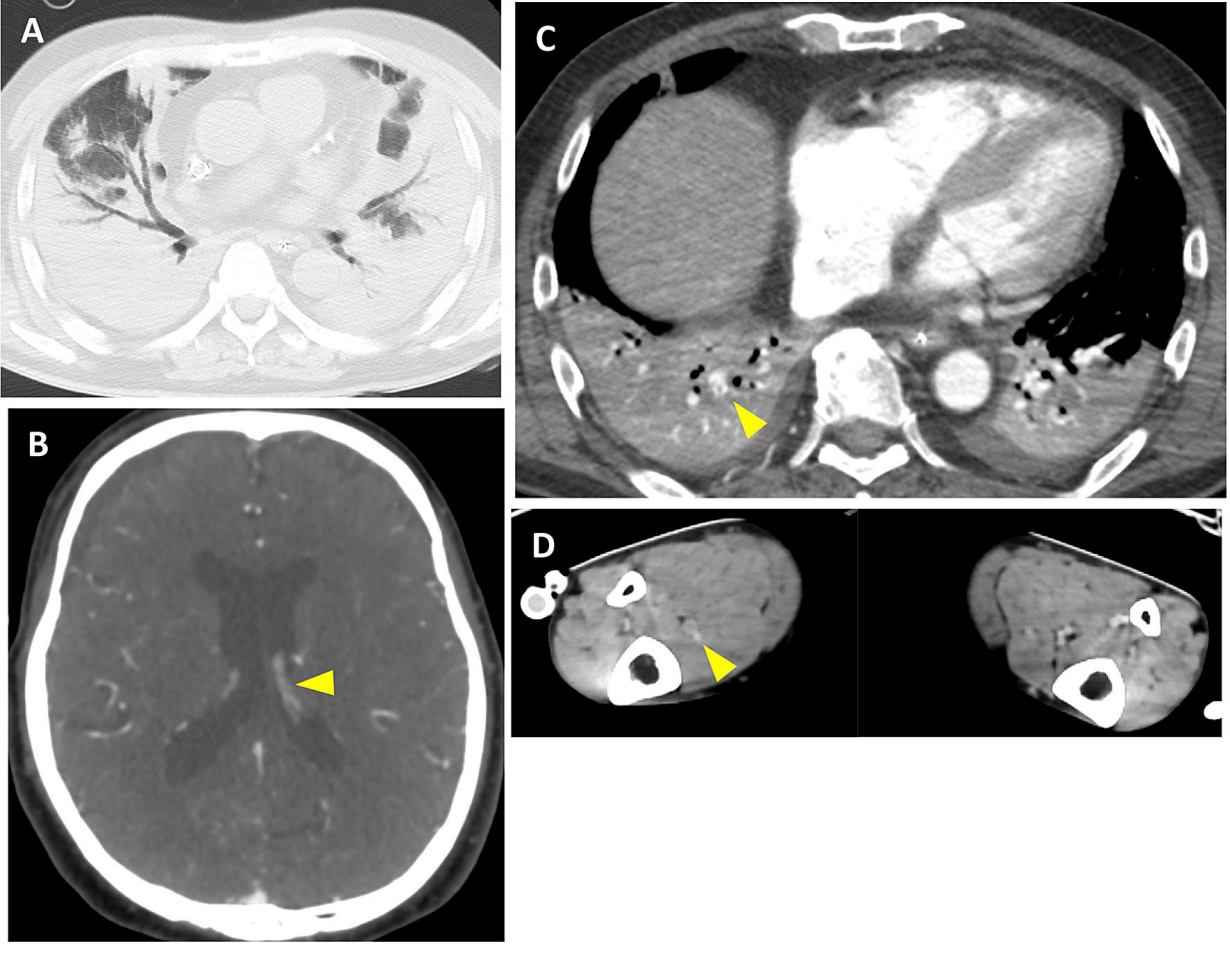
Computed tomography (CT) images. a CT scan showing bilateral and peripheral ground-glass and consolidative pulmonary opacities. CT scan showing b subarachnoid hemorrhage, multiple thrombosis in the c peripheral branch of the right pulmonary artery and d right internal jugular vein and right soleus vein
